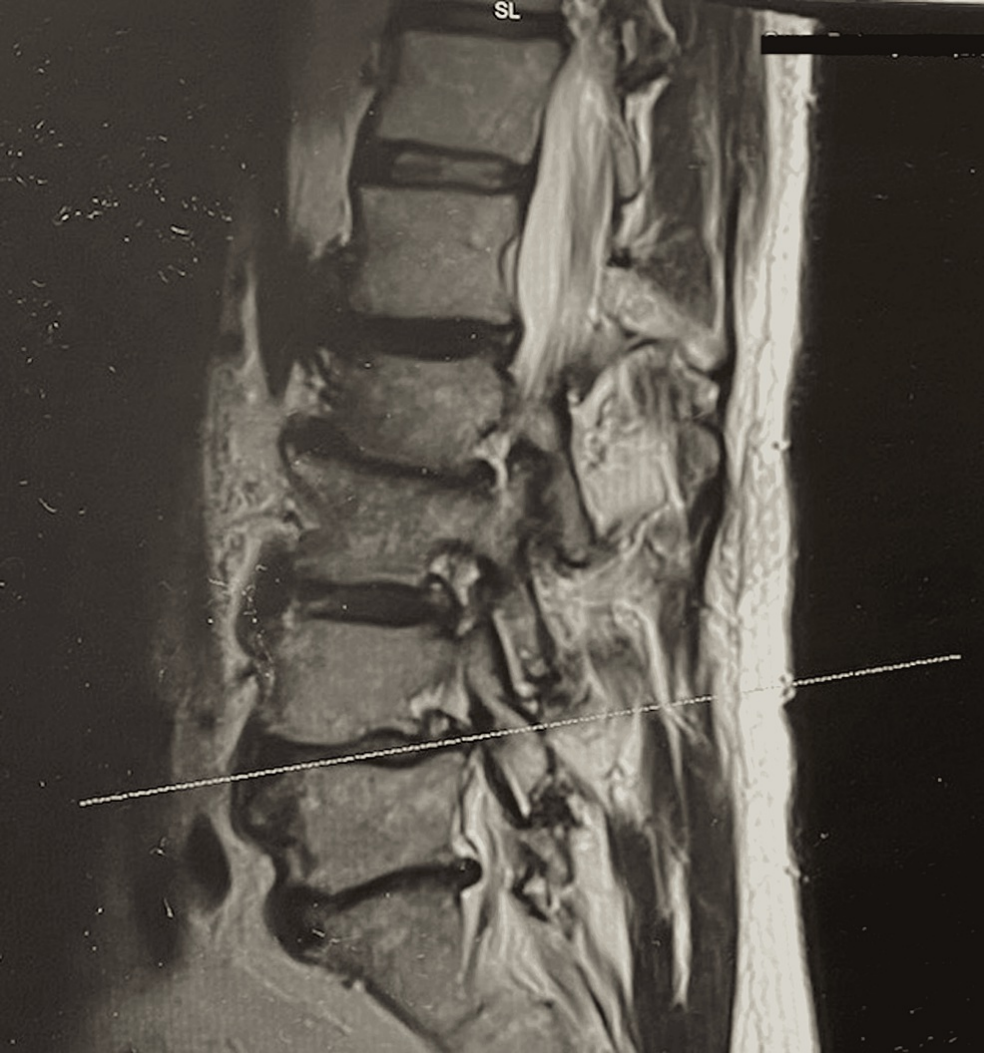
The sagittal MRI image prominently shows severe stenosis at the L4-L5 level, as indicated by the horizontal line, with nerve root impingement as well as L5-S1 foraminal stenosis and nerve root impingement
